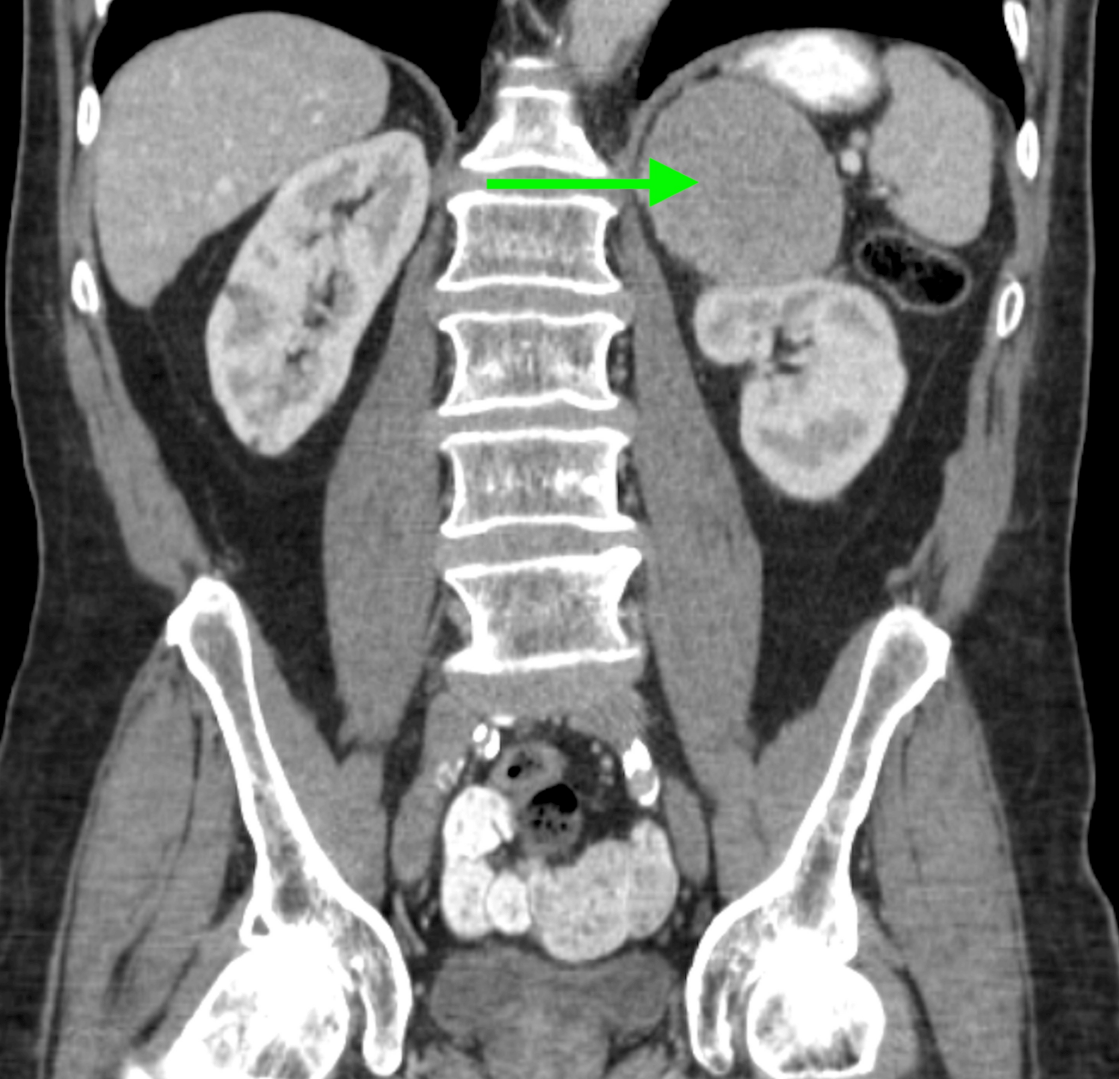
Non-contrast CT scan of the chest and abdomen Green arrow points to the 8 × 11 cm mass in the left upper quadrant, originating from the adrenal gland.
